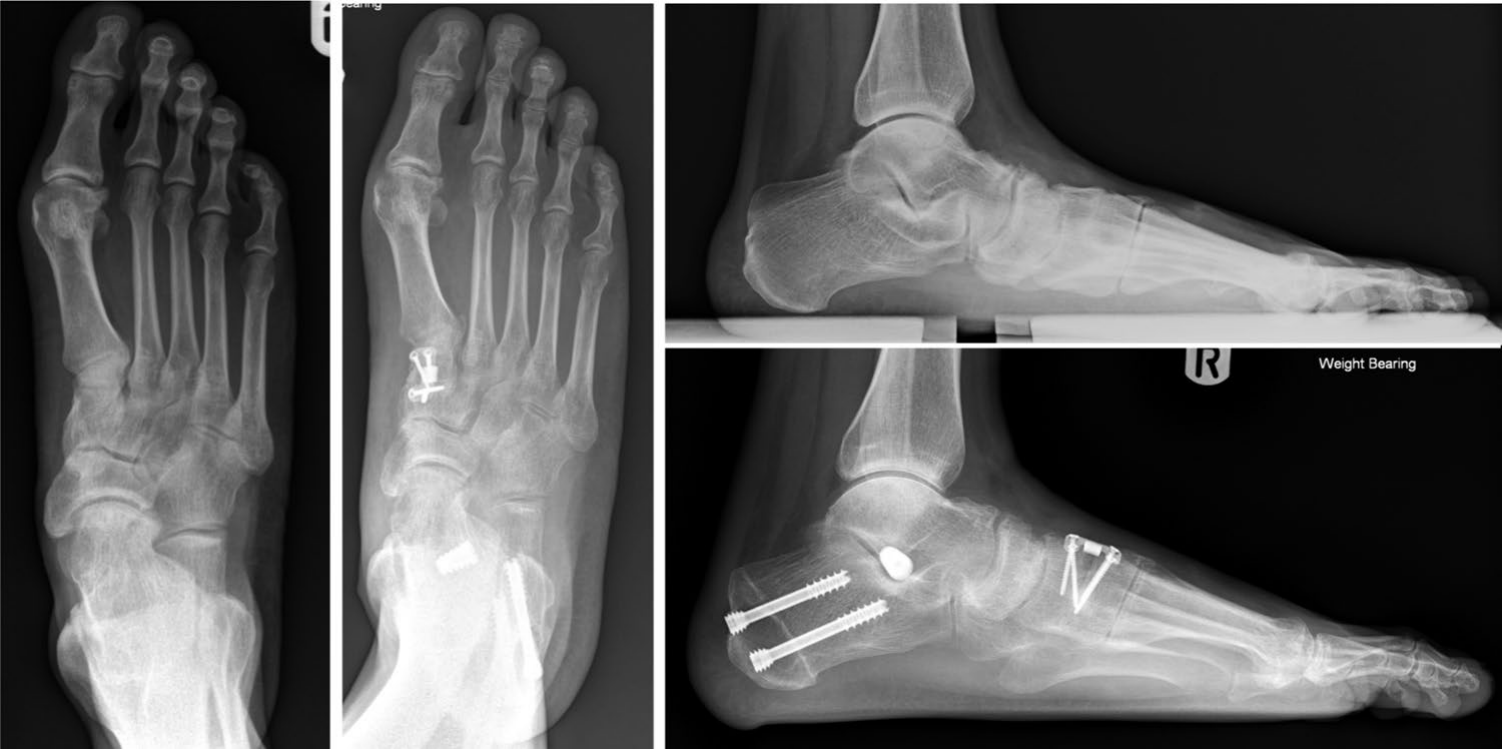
Pre- and post-operative weight-bearing radiographs demonstrating the subtalar arthroereisis implant position just under the lateral shoulder of talus and approximately 50% of the way across the underside of talus
